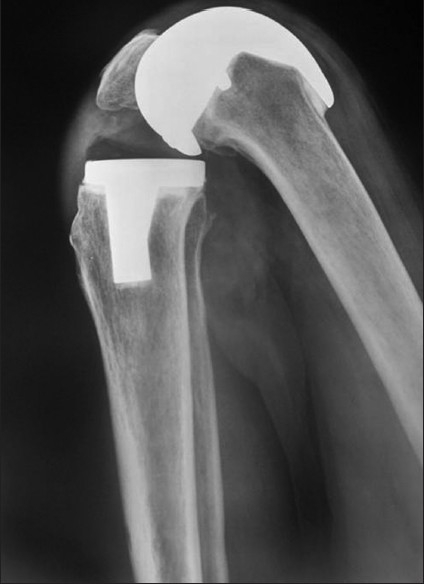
Lateral radiograph of the knee of three year follow up of INDUS knee showing maintaining optimal flexion
